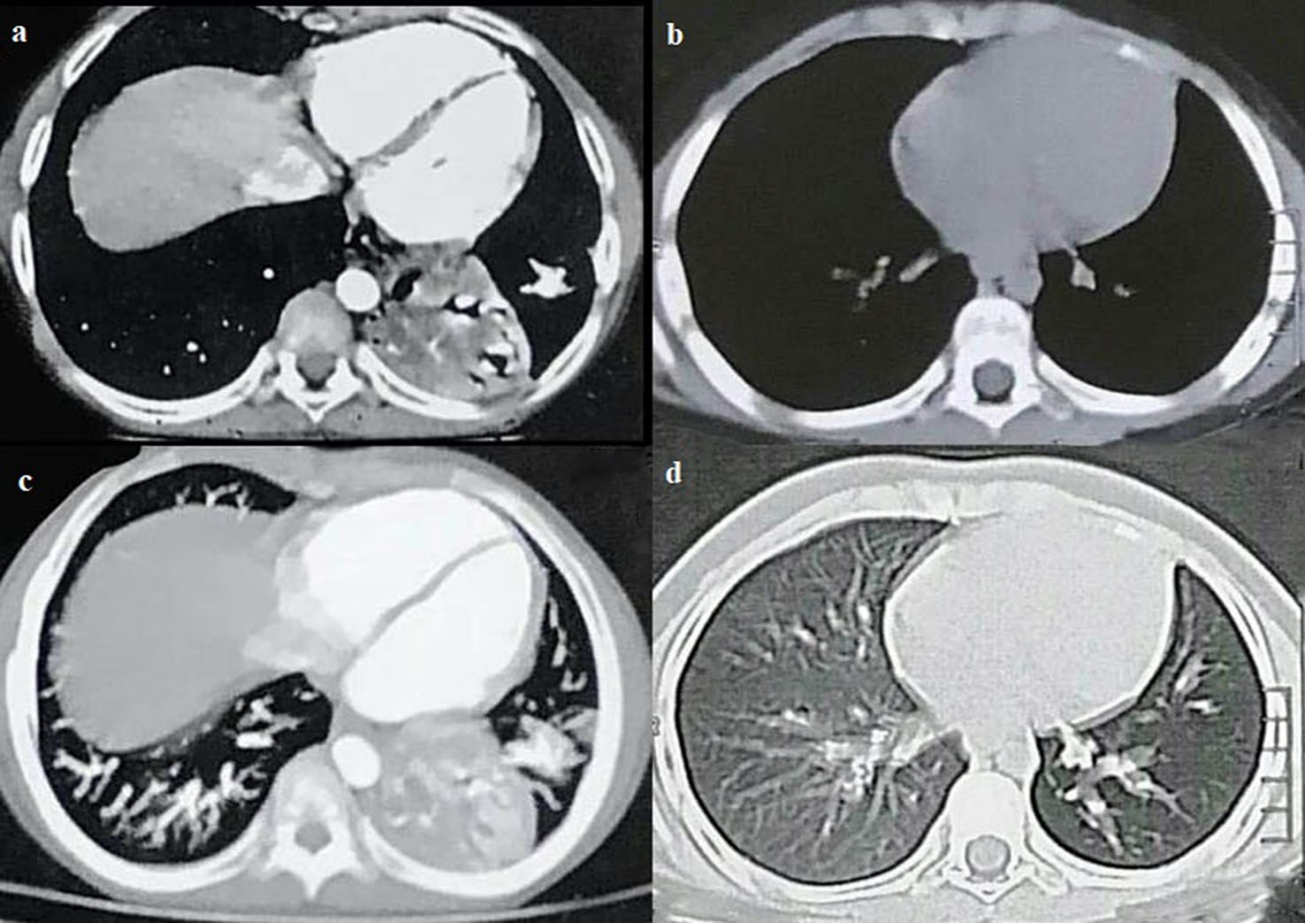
(a) and (b). mediastinal and lung window of CT chest showing left lower sequestrated lobe, (d) and (e). mediastinal and lung window of CT chest 6 months after left lower lobectomy
